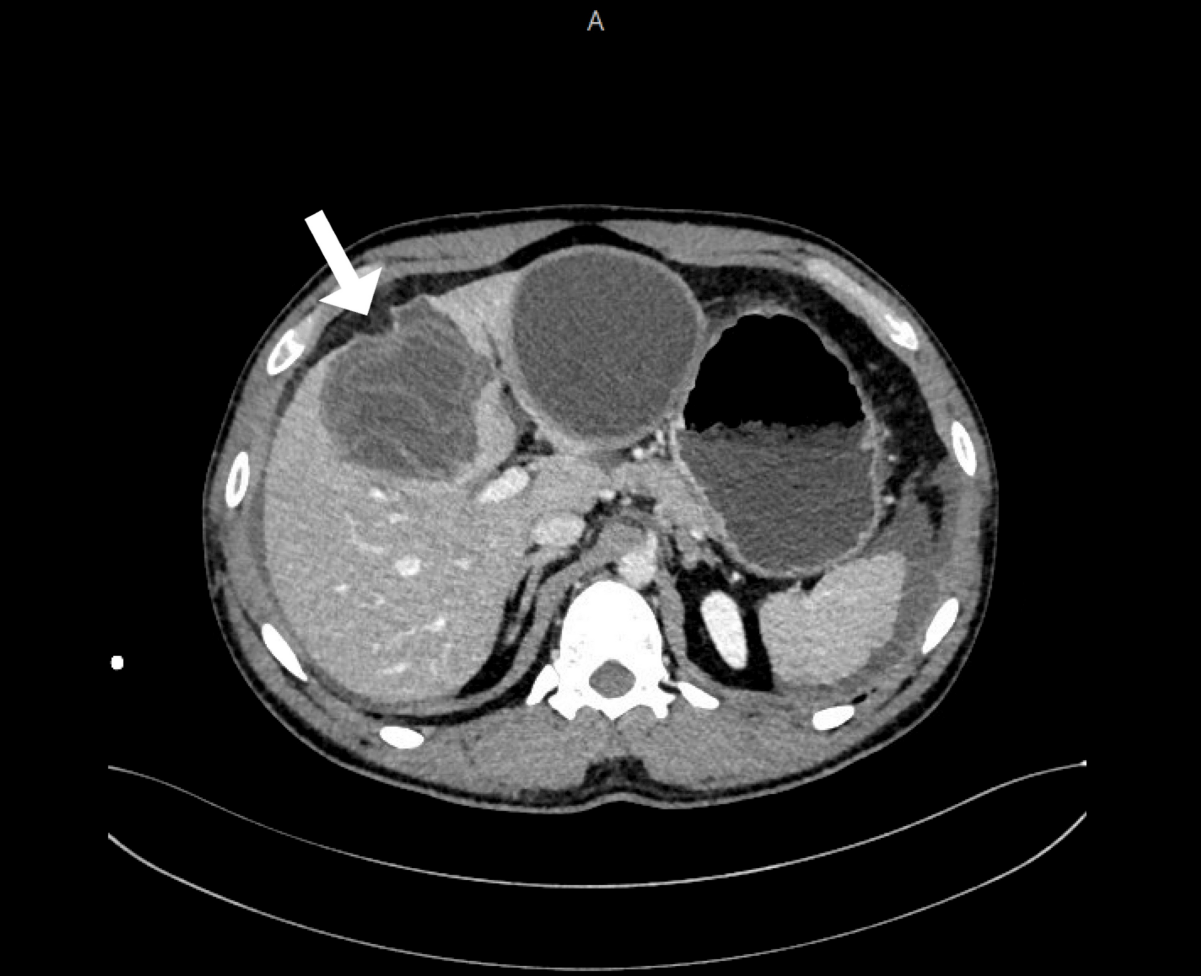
Axial CT image showing multiple hydatid cysts in the liver with one showing signs of rupture (indicated by the arrow)
